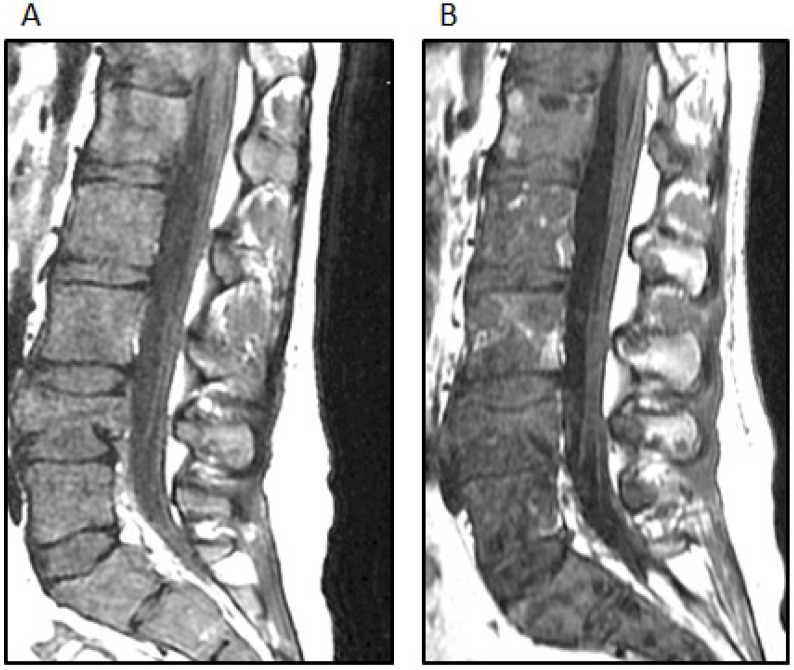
(A) The sagittal STIR (short TI inversion recovery) MRI image demonstrates extensive new osseous metastases with multiple pathologic compression fractures of the lumbar spine; (B) The sagittal T-weighted MRI image demonstrates interval mild improvement in bone marrow signal abnormality after treatment; the fatty replacement of tumor is shown by white arrows.
